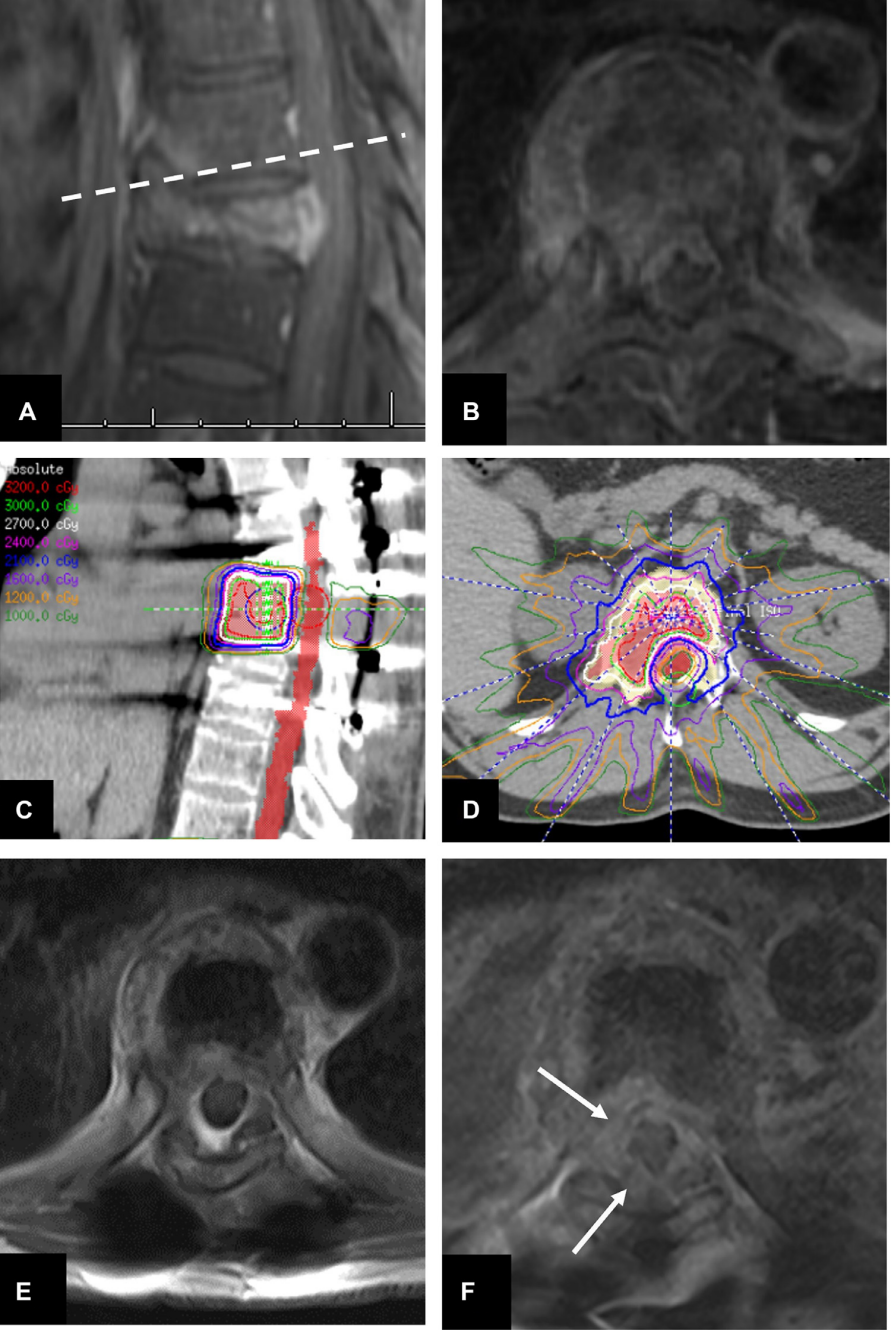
Images from a 50-year-old male with a history of colon cancer metastatic disease to T8 and L1 presenting with progressive bilateral lower extremity weakness during a course of conventional radiation therapy. (A) Postcontrast T1-weighted magnetic resonance imaging showed vertebral compression fracture of T8 with an epidural tumor component (B), causing spinal cord compression. The dashed line corresponds to the level of the axial cuts shown in (E) and (F). Patient underwent partial T8 vertebrectomy and T6 to T10 instrumentation with titanium implants—his neurologic function improved after surgery. (C) and (D), 2700 cGy was delivered in 3 fractions to the same level, from endplate to endplate. (E) Surveillance postcontrast T1-weighted magnetic resonance imaging demonstrating a stable tumor; however, 14 months later, progressive disease (white arrows) was seen in the margins of the irradiated field. Because of the overspread progressive disease, the patient was discharged to hospice care, and died 1 month later.
